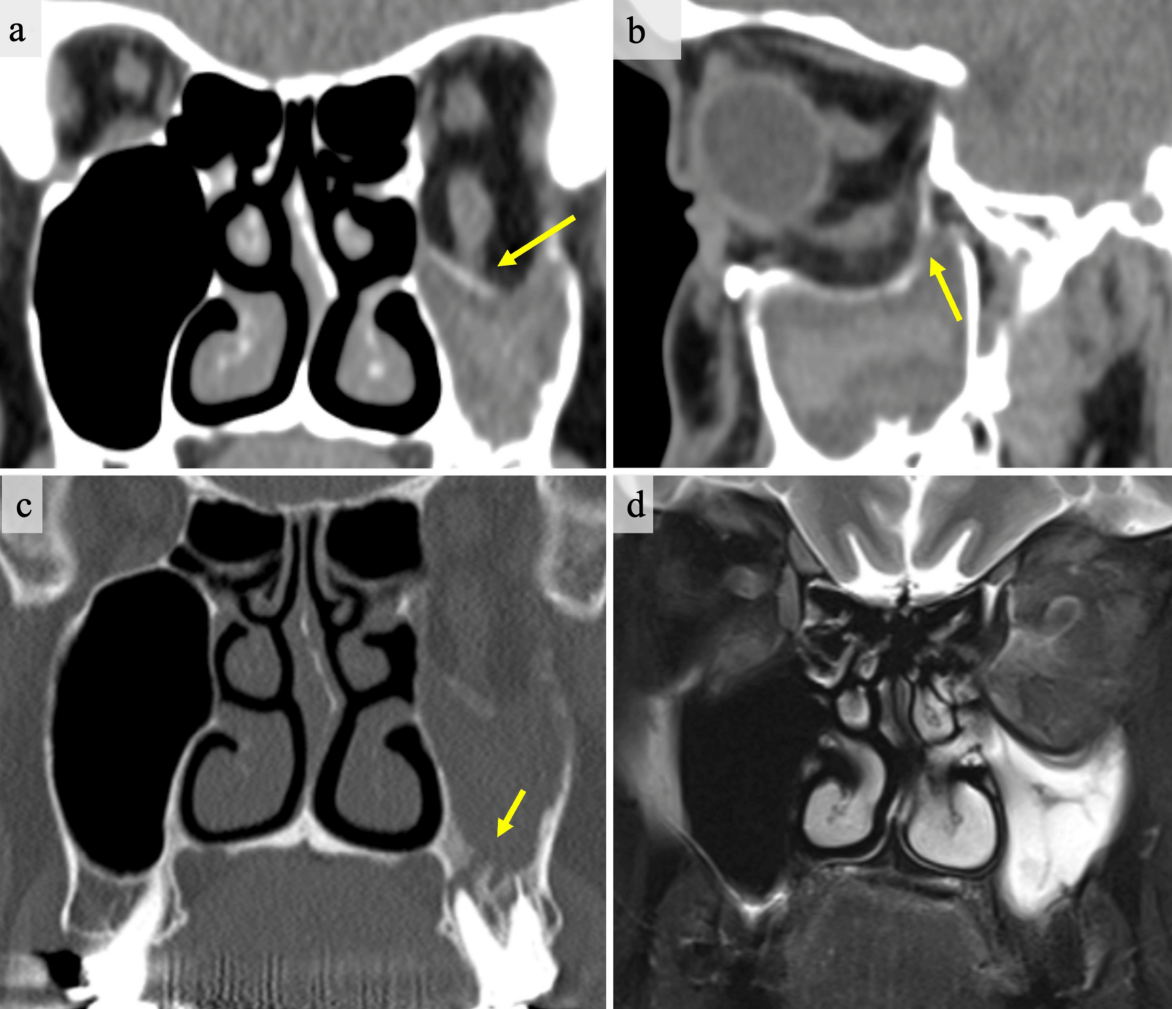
CT and MRI findings at the initial examination in our hospital (two months after the trauma). (a) A coronal CT scan shows significant displacement of the orbital contents, including the inferior rectus muscle. Adhesion of the tissue around the inferior rectus muscle is suspected at the point indicated by the arrow. (b) A sagittal CT scan reveals an extensive orbital floor fracture extending both anteriorly and posteriorly. Additionally, maxillary sinusitis is observed. (c) Findings suggestive of acute inflammation due to odontogenic maxillary sinusitis (the arrow indicates the location). (d) MRI T2-weighted image showing inflammatory findings in the maxillary sinus, with a slightly high signal in the intraorbital fat.
